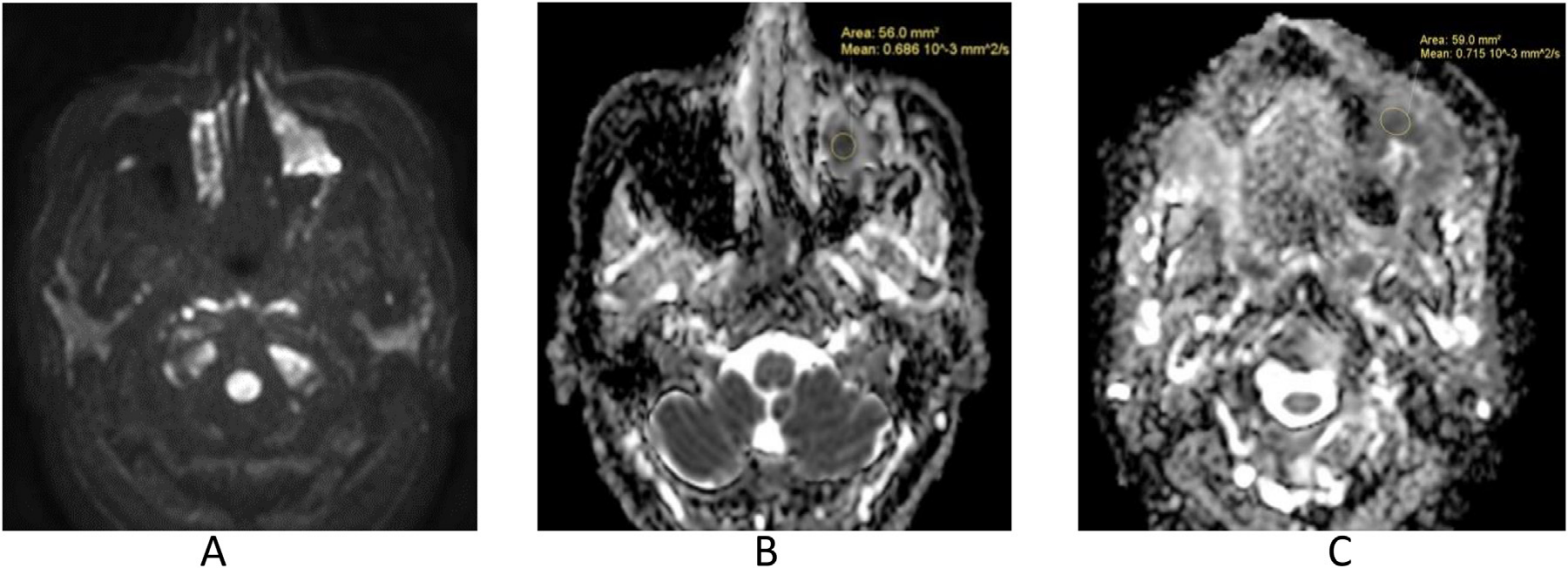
(A-C): DWI (b-value = 800) sequence (A) showed restricted diffusion. ADC map (B, C) revealed a low ADC values of the mass, between 0.6 and 0.7 × 10^−3^ mm^2^/sec: these ADC values suggested the diagnosis of a plasma cells malignancy.
